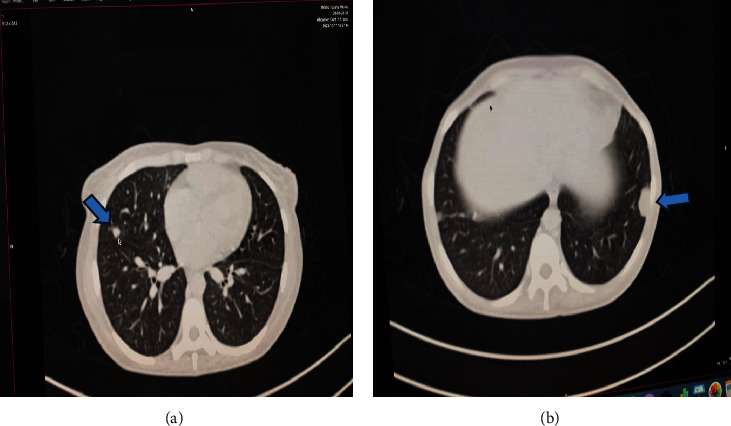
CT scan images showing lung nodules (cannon balls). Reformatted images show lung nodules on the lateral segment of the left lower lobe, (a) coronal view, with a blue arrow showing the lung nodule. (b) The sagittal view shows a pleural-based lung nodule (blue arrow).
